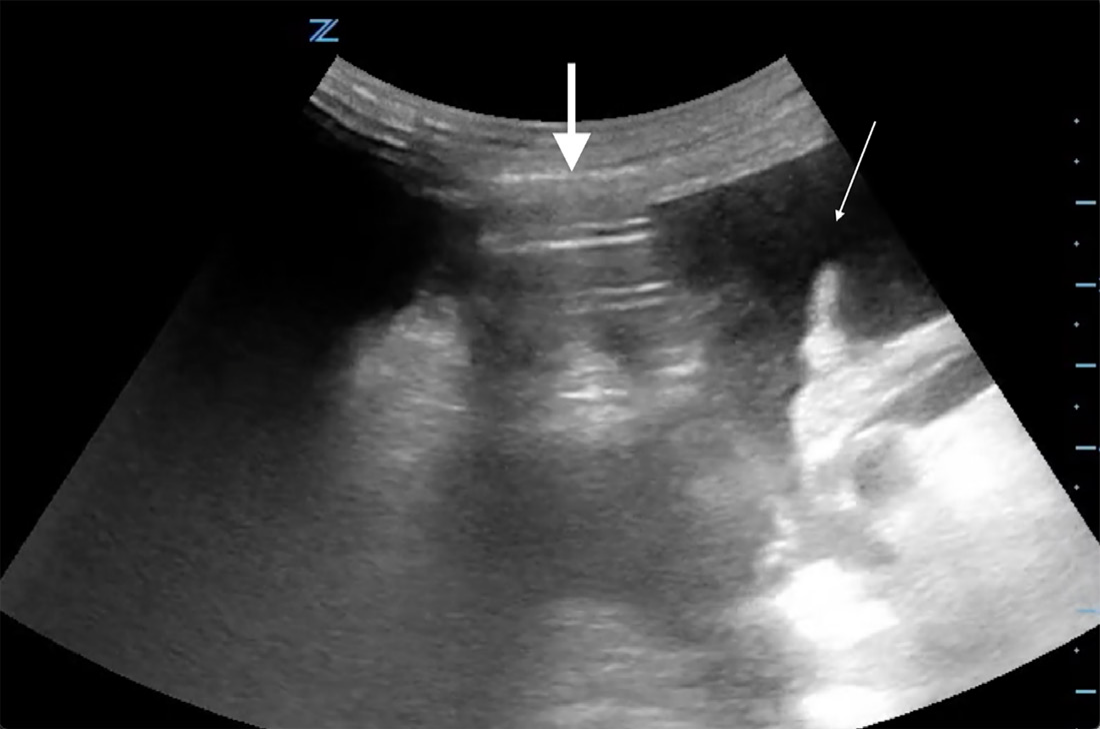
Abdominal ultrasound from Case 1 showing free intraperitoneal fluid (thin arrow) as well as free intraperitoneal air (thick arrow) as demonstrated by the enhanced peritoneal stripe sign and reverberation artifact.
